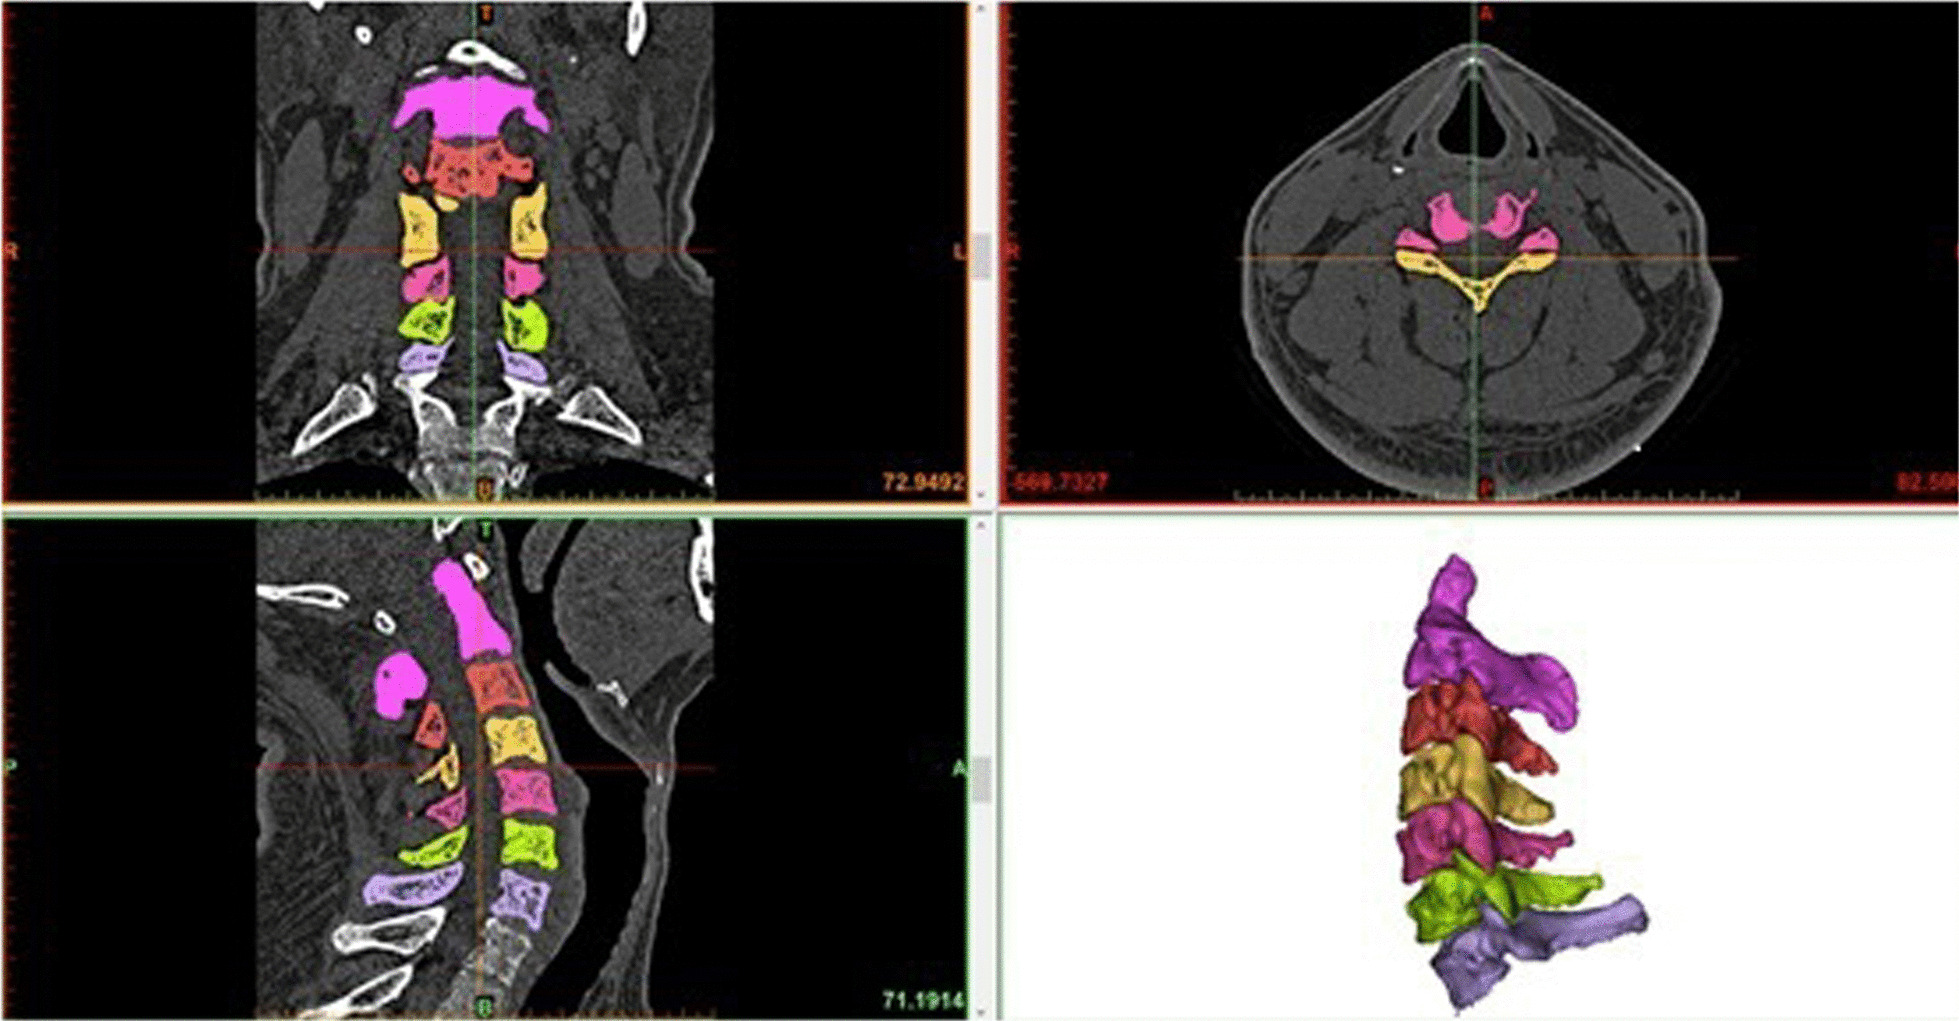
CT data file processingReverse reconstruction of the 3D solid modelThe STL file was imported into Geomagic software (Geomagic 2017, USA), and inverse reconstruction processing comprising denoising, polishing, and smoothing was performed to form a 3D image that was saved in IGES format (Fig. 2). Three-dimensional computer-aided design modeling software (Unigraphics NX12.0, Siemens PLM software) was used to establish structural models of the cortical bone, cancellous bone, endplates, nucleus pulposus, fibrous rings, and facet joints of the vertebral bodies, and a 3D solid model of C3–C7 was formed and saved in IGES format (Fig. 3).
